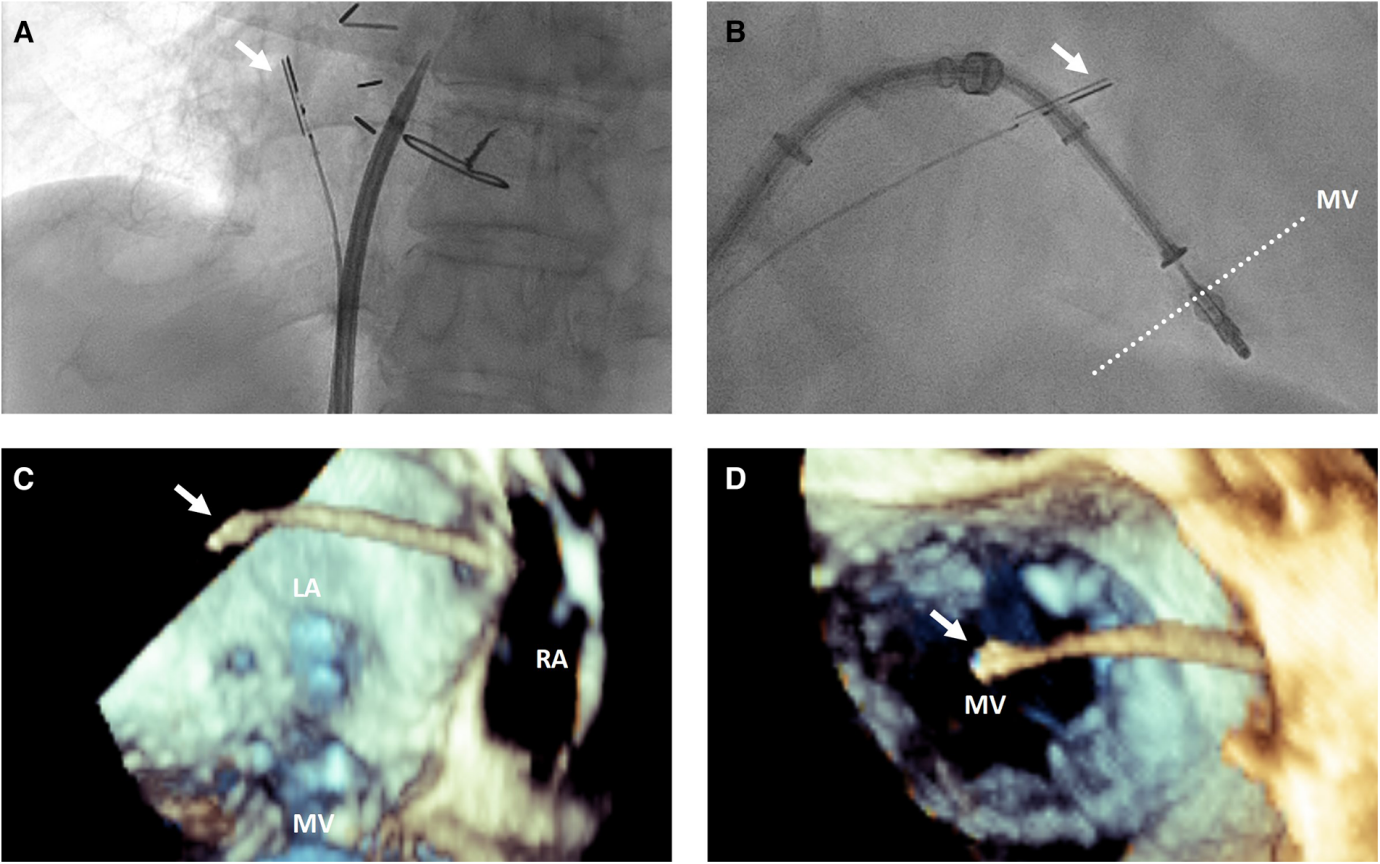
3-dimensional intracardiac echocardiography probe positioning for transcatheter mitral interventions. (A) Fluoroscopic view demonstrating the intracardiac echocardiography probe (white arrow) positioning with posterior flexion relative to a trans-septal puncture system to image the interatrial septum for trans-septal puncture; (B) fluoroscopic and 3-dimensional transesophageal echocardiographic views from posterior looking anterior (C) and from a surgeon's view above the mitral valve from the left atrium (D) demonstrating the intracardiac echocardiography probe (white arrow) positioning relative to the mitral valve for intraprocedural guidance of a transcatheter edge-to-edge repair. LA, left atrium; RA, right atrium; MV, mitral valve.
